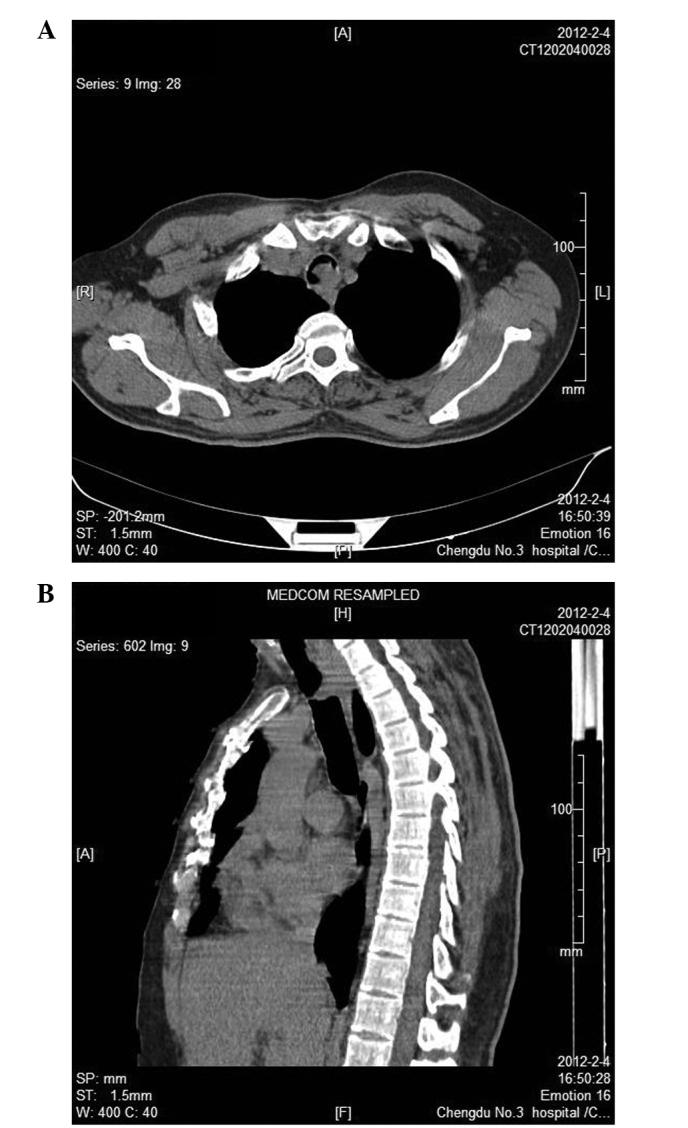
Computed tomograms of homogenous mass intratrachea at the layer of the superior border of the manubrium: (A) Transverse section and (B) median sagittal section of the chest.
